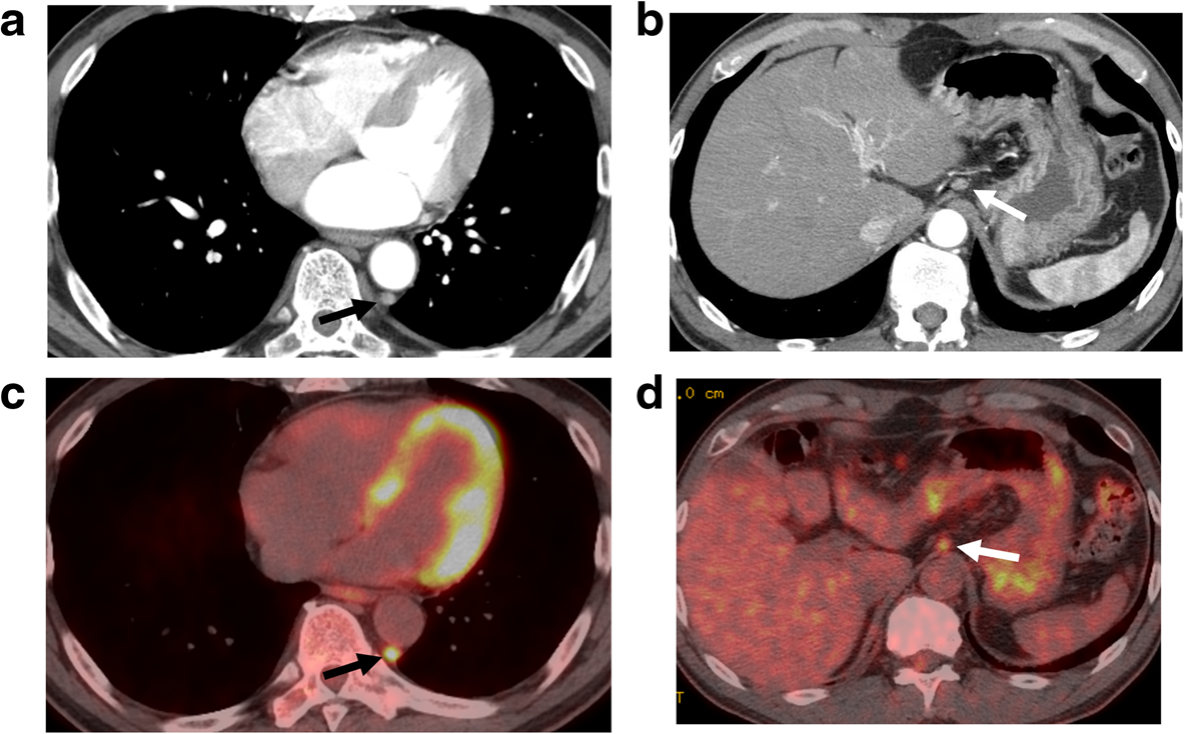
The computed tomography findings. Findings of the metastatic mediastinal (a, c) and abdominal (b, d) lymph nodes. Computed tomography (CT) showing a swollen lymph node, 0.8 cm in size, in the retroaortic area and accumulation of F-deoxyglucose (FDG) in FDG positron emission tomography (FDG-PET) (black arrow). CT scan and FDG-PET imaging also showed a swollen lymph node with FDG accumulation in the perigastric node along the left gastric artery (white arrow).
